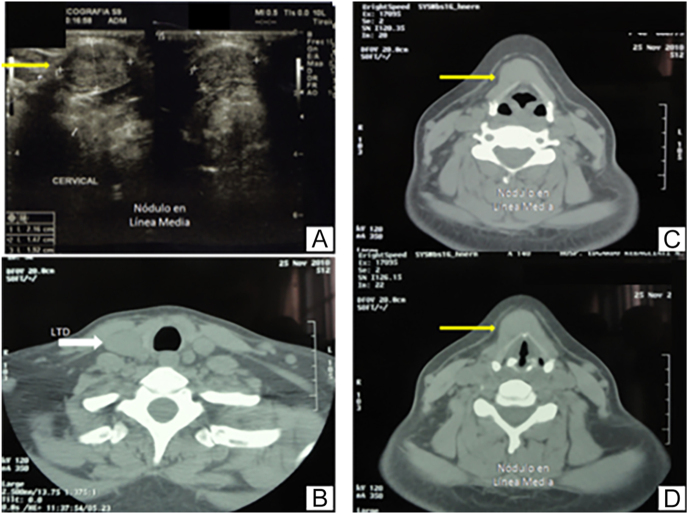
Cervical ultrasound showing a prehyoid nodule (A). Cervical CT demonstrating a homogeneous prehyoid nodule and the absence of nodules in the thyroid gland (B, C, D). In image (A), the arrow points to the cyst on the ultrasound; in image (B), the arrow points to the right lobe without nodules on the CT scan; and in images (C and D), the arrows point to the thyroglossal duct cyst on the CT scan.
